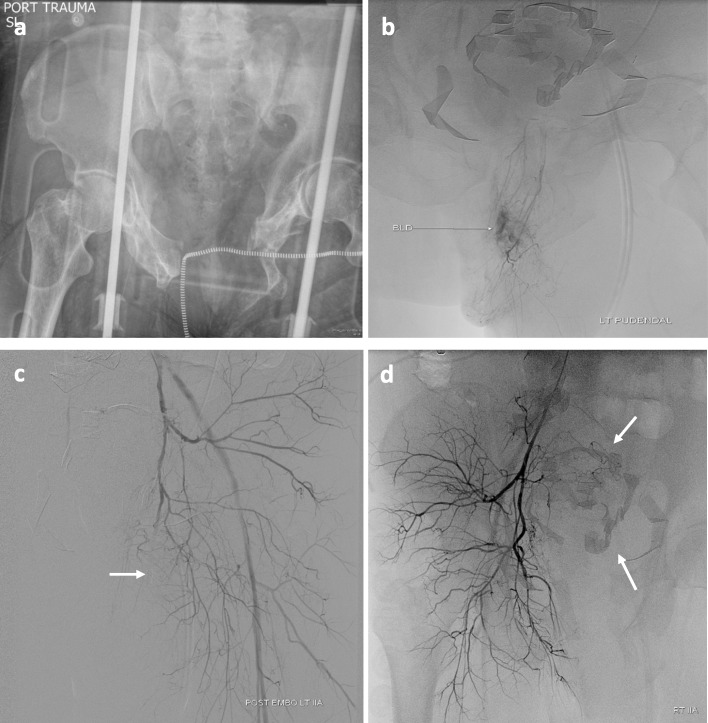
Patient 1 Initial angioembolization procedure via a trans-radial approach: AP Pelvis radiograph showing open book pelvic fractures (a). Superselective left pudendal artery angiogram (b). Follow-up left internal iliac angiogram showing coil occluded (arrow) left pudendal artery, reflux into the external iliac artery, and no other signs of hemorrhage in the left hemi-pelvis (c). Negative right internal iliac angiogram also showing reflux into the external iliac artery. Note lap sponges in the mid pelvis (arrrows) (d)
